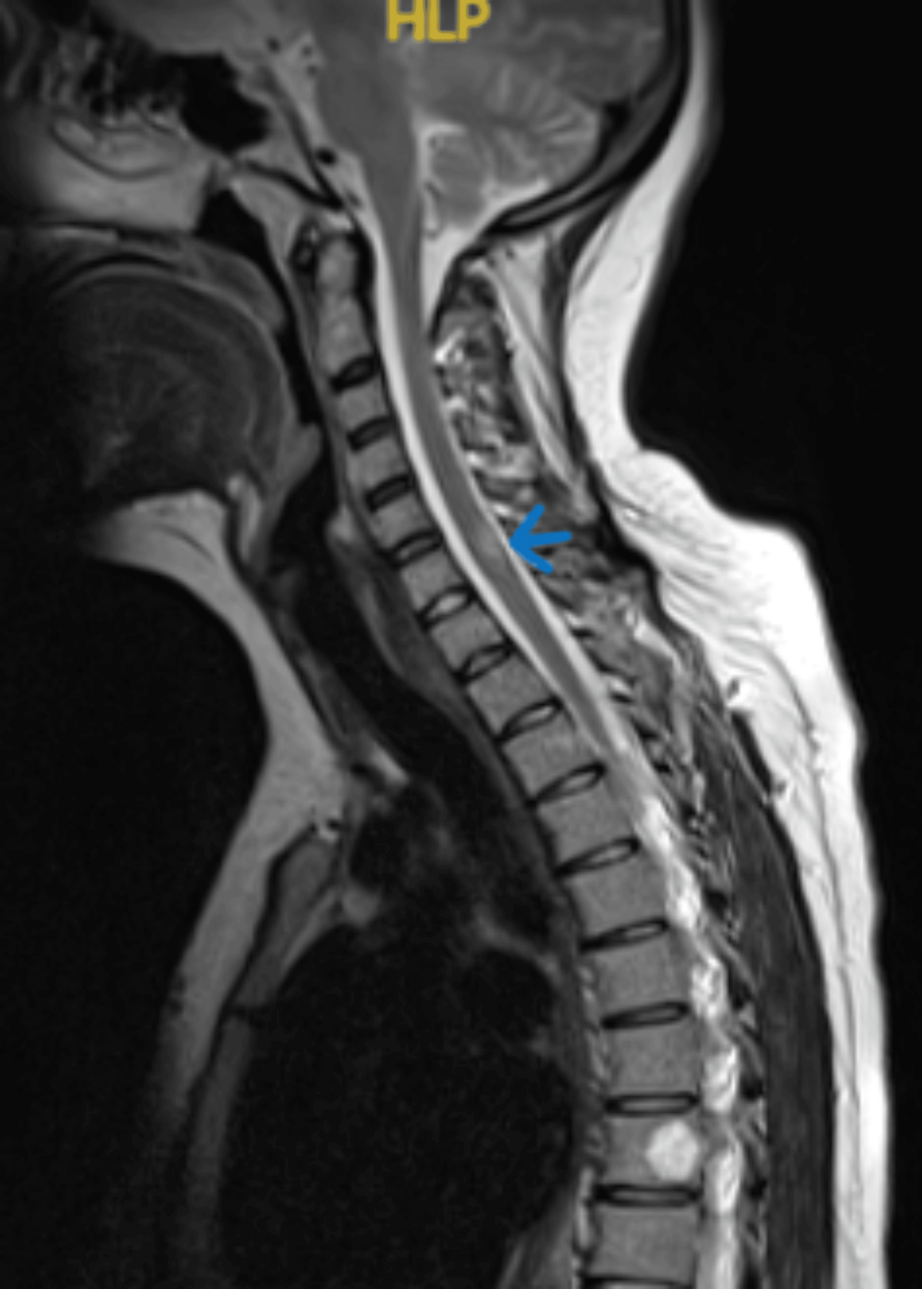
T2-weighted MRI of the cervical spine showing a hyperintense lesion at the C3-C4 level (indicated by the blue arrow), suggestive of demyelination
